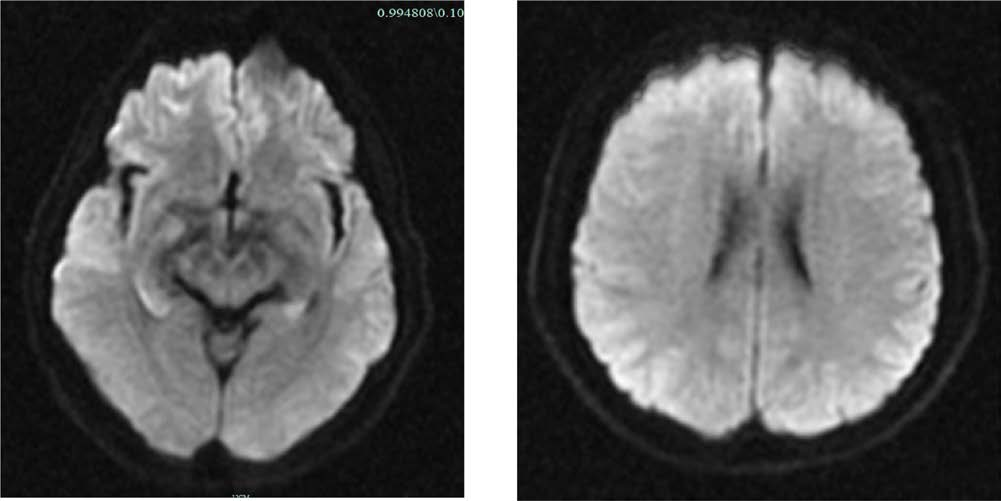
Craniocerebral MRI (DWI): cerebral tissue has no obvious signs of infarction. MRI = magnetic resonance imaging.
